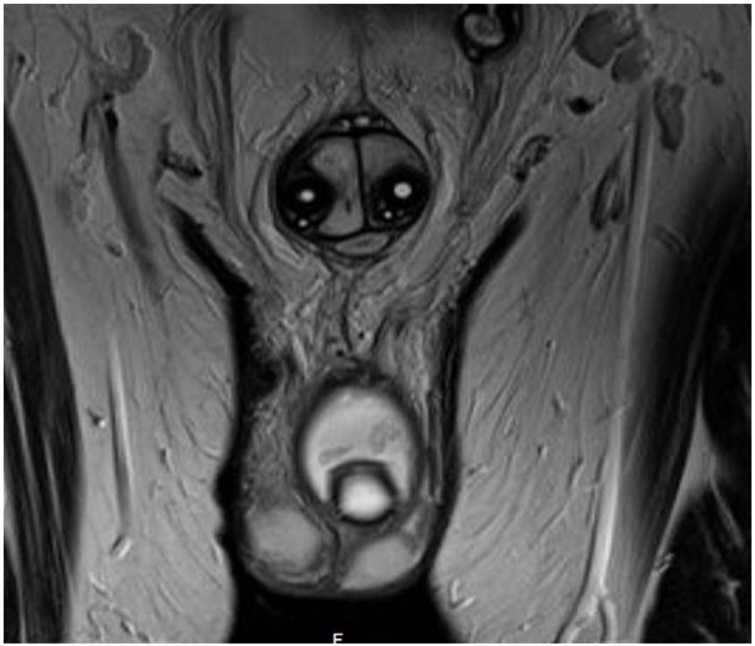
Pelvic MRI showing a scrotal collection around the pump.
